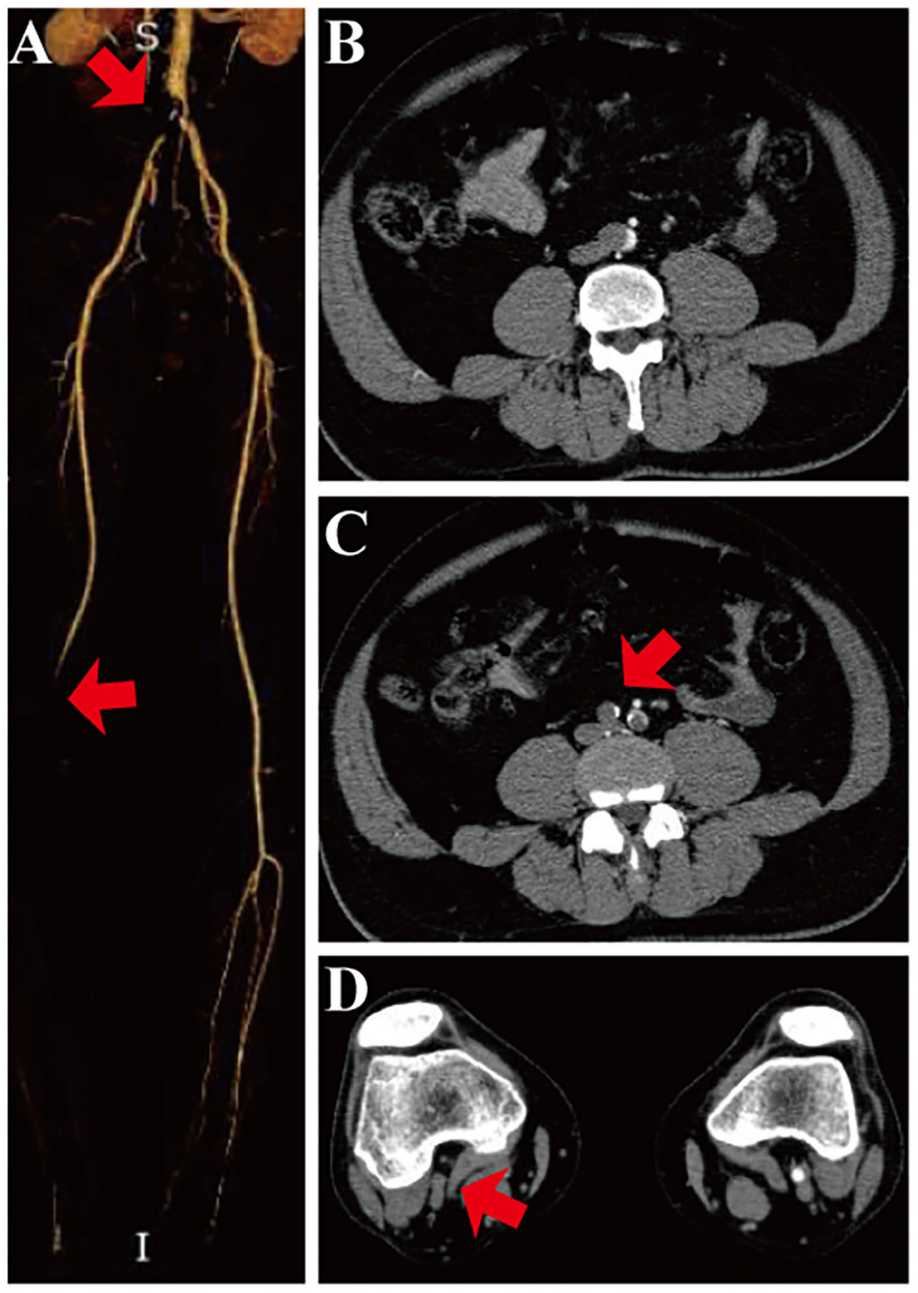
Preoperative CTA scan of the abdominal aorta and peripheral artery. (A) Three-dimensional CTA scan shows embolism (red arrow) in the inferior segment of the abdominal aorta, bilateral common iliac artery, and the right popliteal artery. (B) Cross-section of CTA shows embolism in the inferior segment of the abdominal aorta. (C) Cross-section of CTA shows embolism in the bilateral common iliac artery (red arrow). (D) Cross-section of CTA shows embolism in the right popliteal artery (red arrow).
